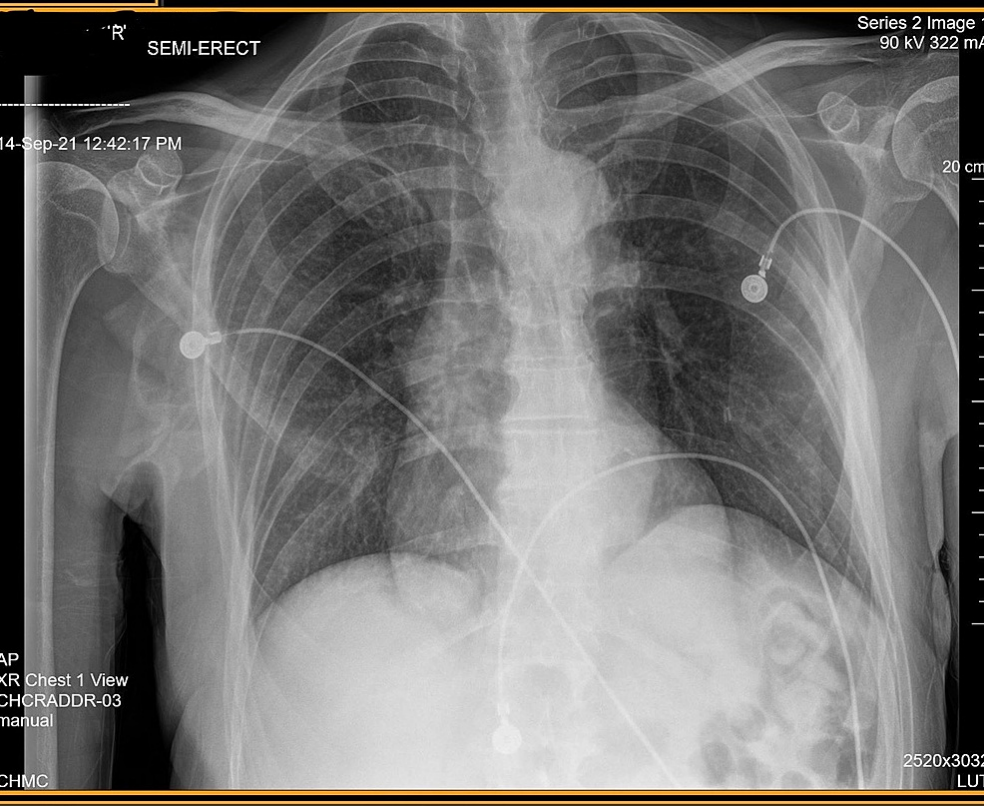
Single front-view X-ray of the chest with no evidence of acute disease and a calcified aorta consistent with atherosclerotic disease.
